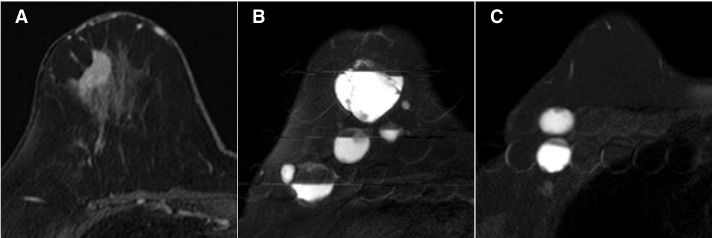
Contrast-enhanced MRI of the breast. (A) A 27 × 15-mm early darkened mass lesion extending toward the nipple in the lateral superior region of the right breast was observed, which was considered to be consistent with the calcified area on the mammogram and the hypoechoic area noted on the ultrasound. (B) Numerous cystic masses are present in the nipple to the lateral inferior region, with an internal T2 high-signal fluid formation. (C) Similar cystic masses are present in the axilla.
